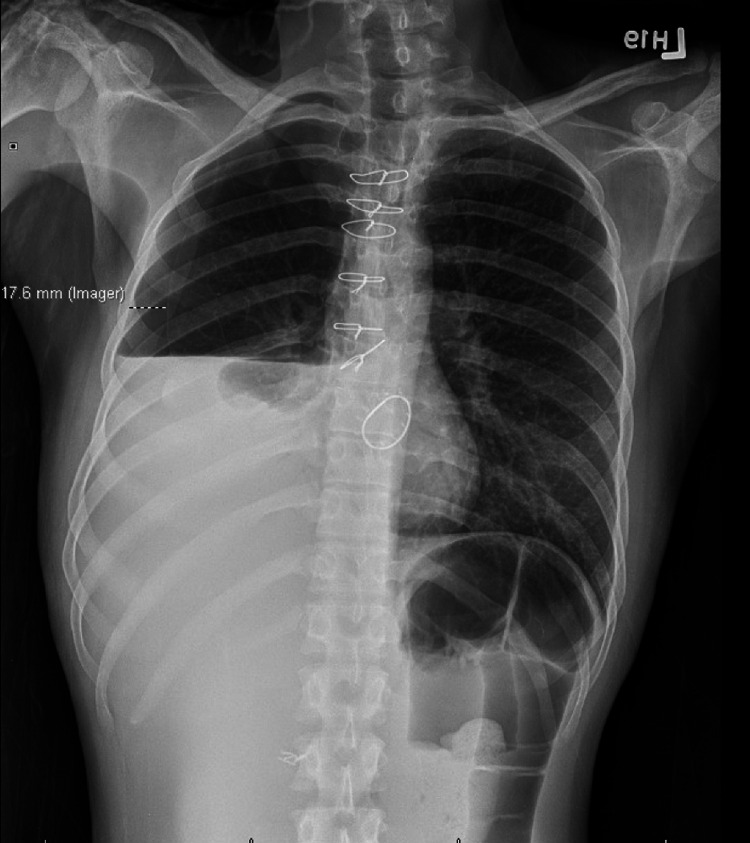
Right pleural effusion on chest X-ray
